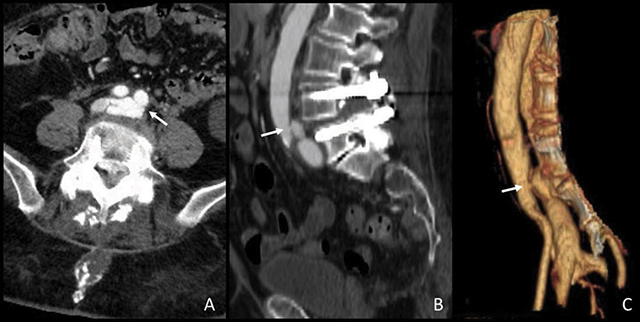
Angio-CT revealing an arteriovenous fistula involving the left common iliac vessels.
